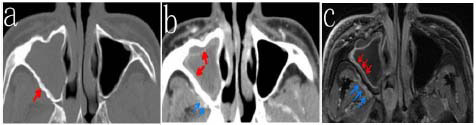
Plain CT scan of the bone window (a) showing right maxillary sinusitis with significant cortical bone remodeling (red arrow). Contrast-enhanced soft tissue window (b) showing mucosal enhancement (red arrows) and increased vascularity (blue arrows). Contrast-enhanced MR image (c) showing thickening and significant enhancement of the mucosa in the right maxillary sinus (red arrows), with a slightly enhanced submucosal layer, indicating mucoperiosteal layering and increased vascularity (blue arrows).
